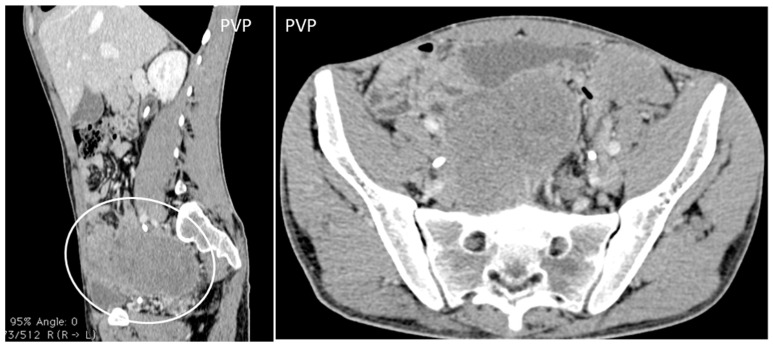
A 26-year-old patient coming to medical attention for abdominal tenderness and sudden weight loss. Contrast enhanced sagittal and axial CT scan shows the characteristic CT findings of desmoplastic small round cell tumor (DSRCT): bulky multiple, soft-tissue masses with modest enhancement, and omental, serosal, and rectovesical involvement with rare calcifications, invading the anterior abdominal wall.
